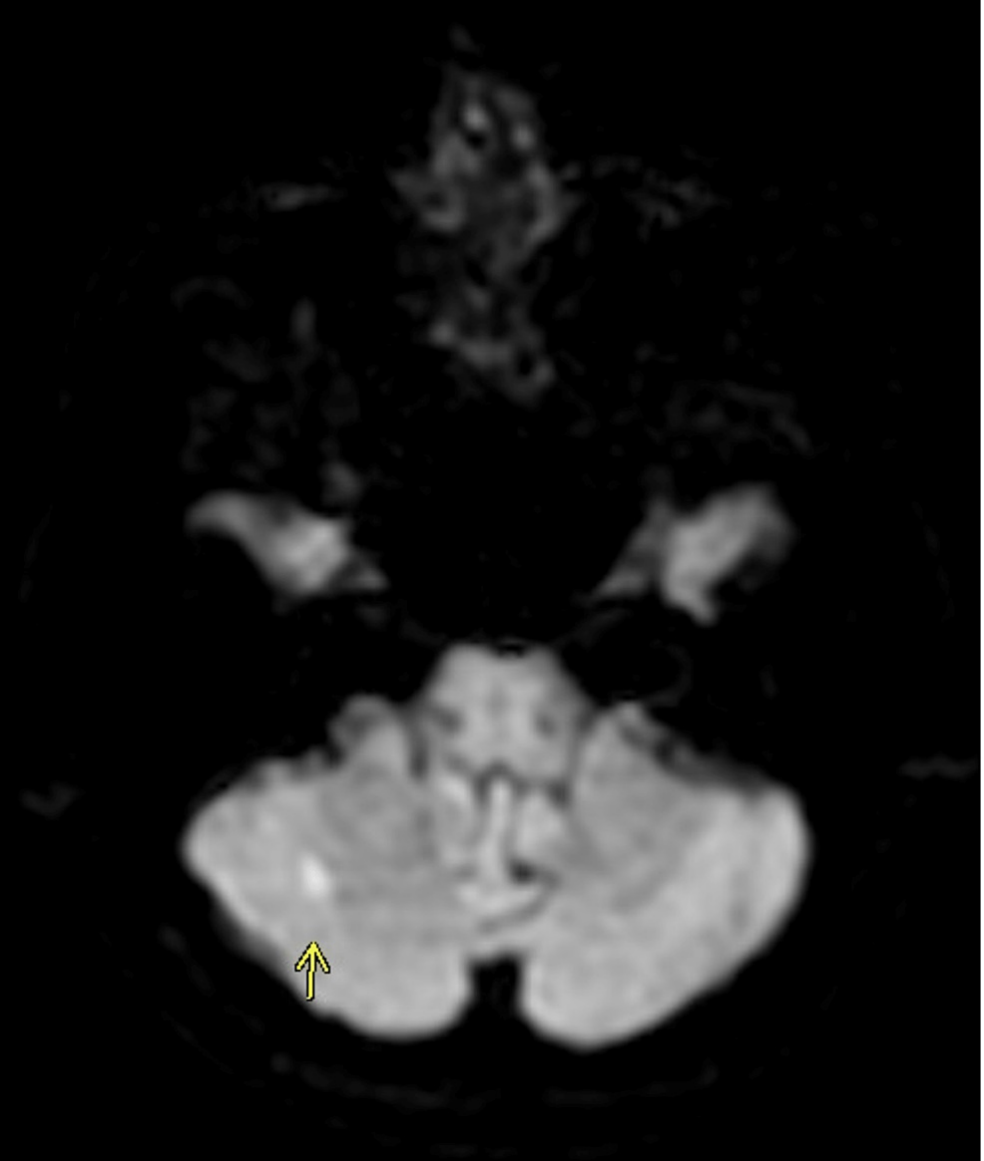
Diffusion-weighted magnetic resonance imaging (DWI-MRI) showing cerebellar infarct (arrow)
